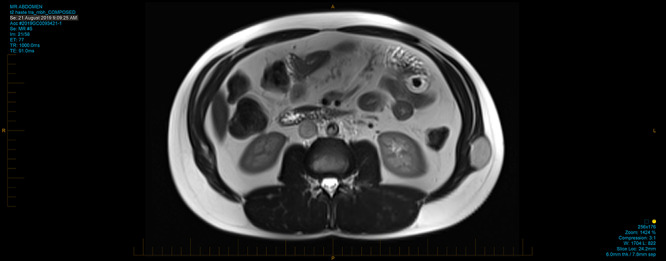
Axial section of abdominal T2 weighted MRI showing hyperintense mass within the left external oblique muscle.
